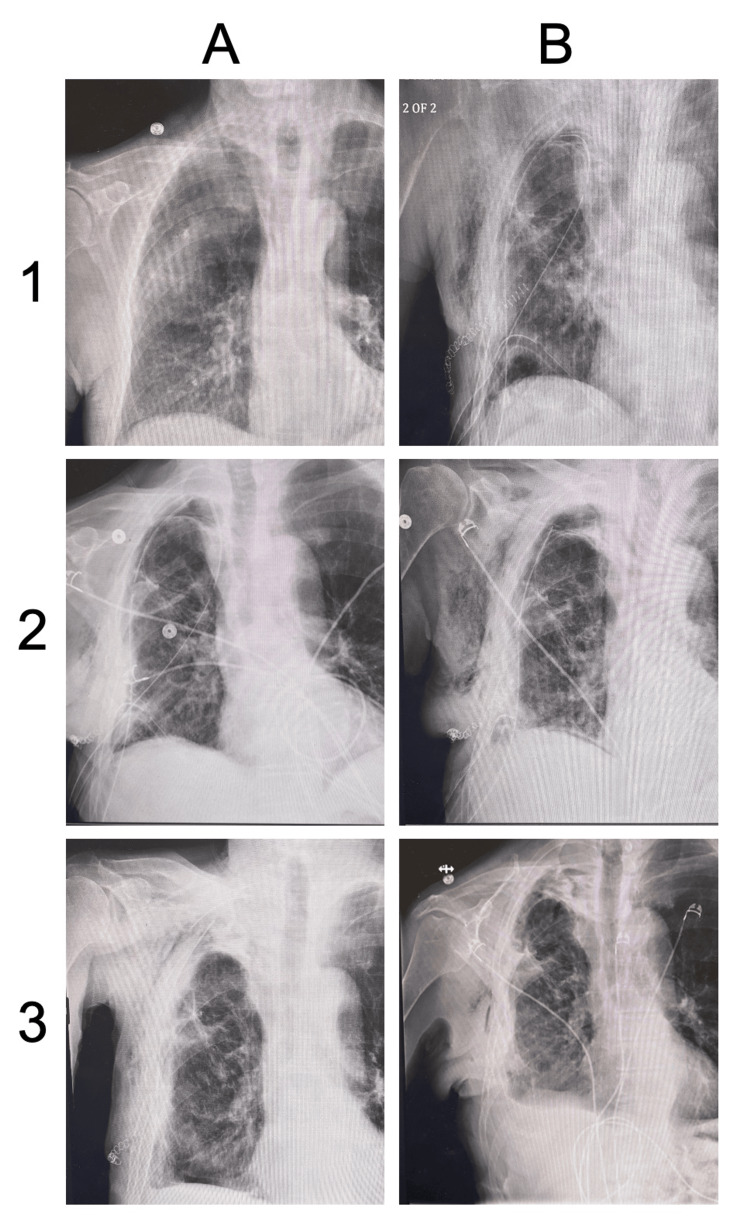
Chest radiographs of serial fibrin glue pleurodesis Plain posterior to anterior chest radiographs showing pre-surgical thoracotomy with wedge resection of the right upper lobe lung mass (1A). 1B showing postoperative changes following redo thoracotomy with a sixth rib resection and addition of three chest tubes. Serial fibrin glue pleurodesis resulting in progressive resolution of apical pneumothorax and subcutaneous emphysema (2A-3B). Note the posterior apical chest tube removal on postoperative day (POD) 6 (2B) and removal of remaining chest tubes on PODs 9 and 10 (3A and 3B, respectively). Pleurodesis material can be seen filling residual pleural space in 3B, resulting in the resolution of the air leak.
